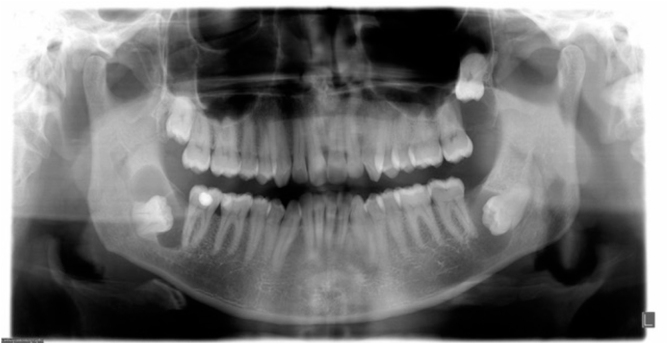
Panoramic radiograph showing cystic area around the unerupted teeth in three quadrants.
